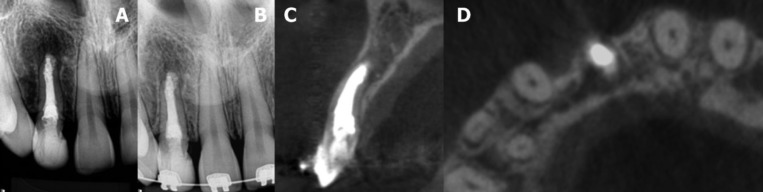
A. The patient returned for clinical and radiographic examinations after six (a) and B. fourteen months. C. Sagittal and D. axial CBCT images of a new scan performed after fourteen months, showing advanced healing and periapical repair.
